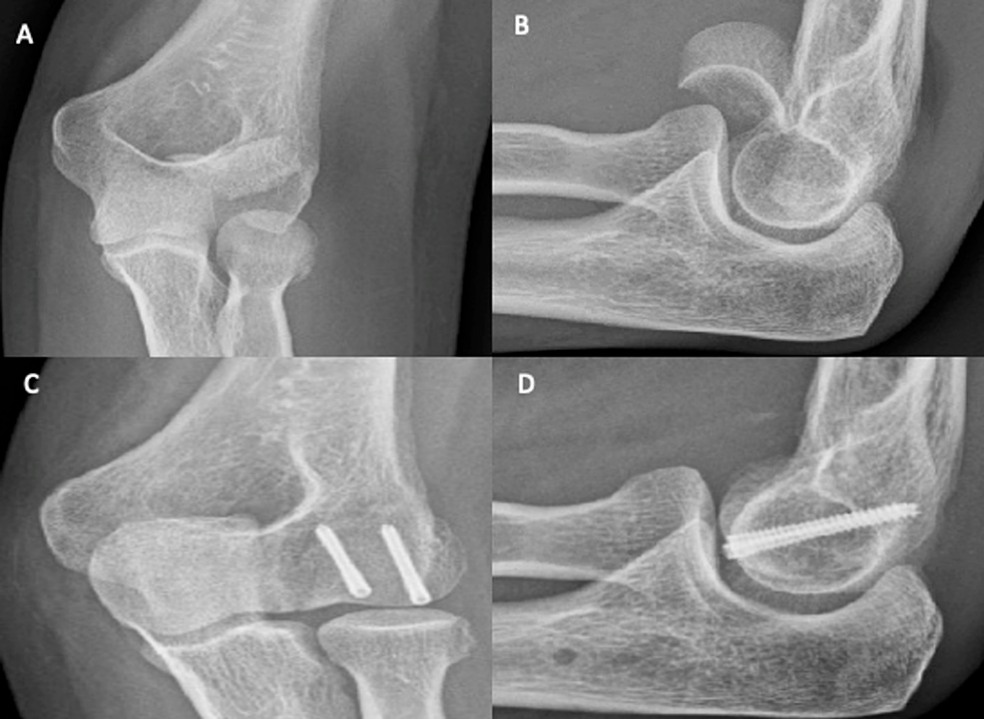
Plain elbow X-rays of case 1. Figure 1A, 1B shows anteroposterior and lateral views, respectively, of a type IV capitellum fracture. There is a large capitellum fragment, with the involvement of the lateral half of the trochlea. Figure 1C, 1D shows anteroposterior and lateral views, respectively, of the elbow after ORIF. The fracture has been successfully reduced using two Mini Acutrak® screws. ORIF: open reduction and internal fixation
